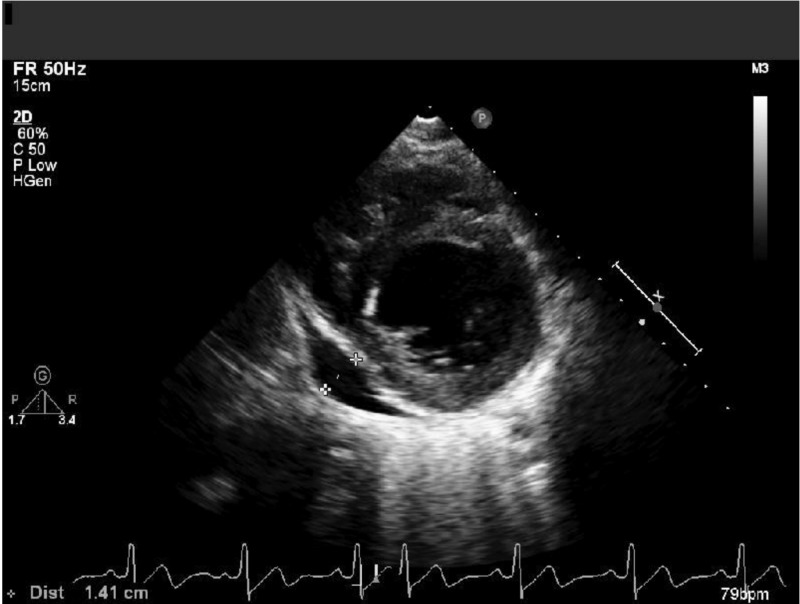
Short axis parasternal view showing a moderate-sized pericardial effusion
